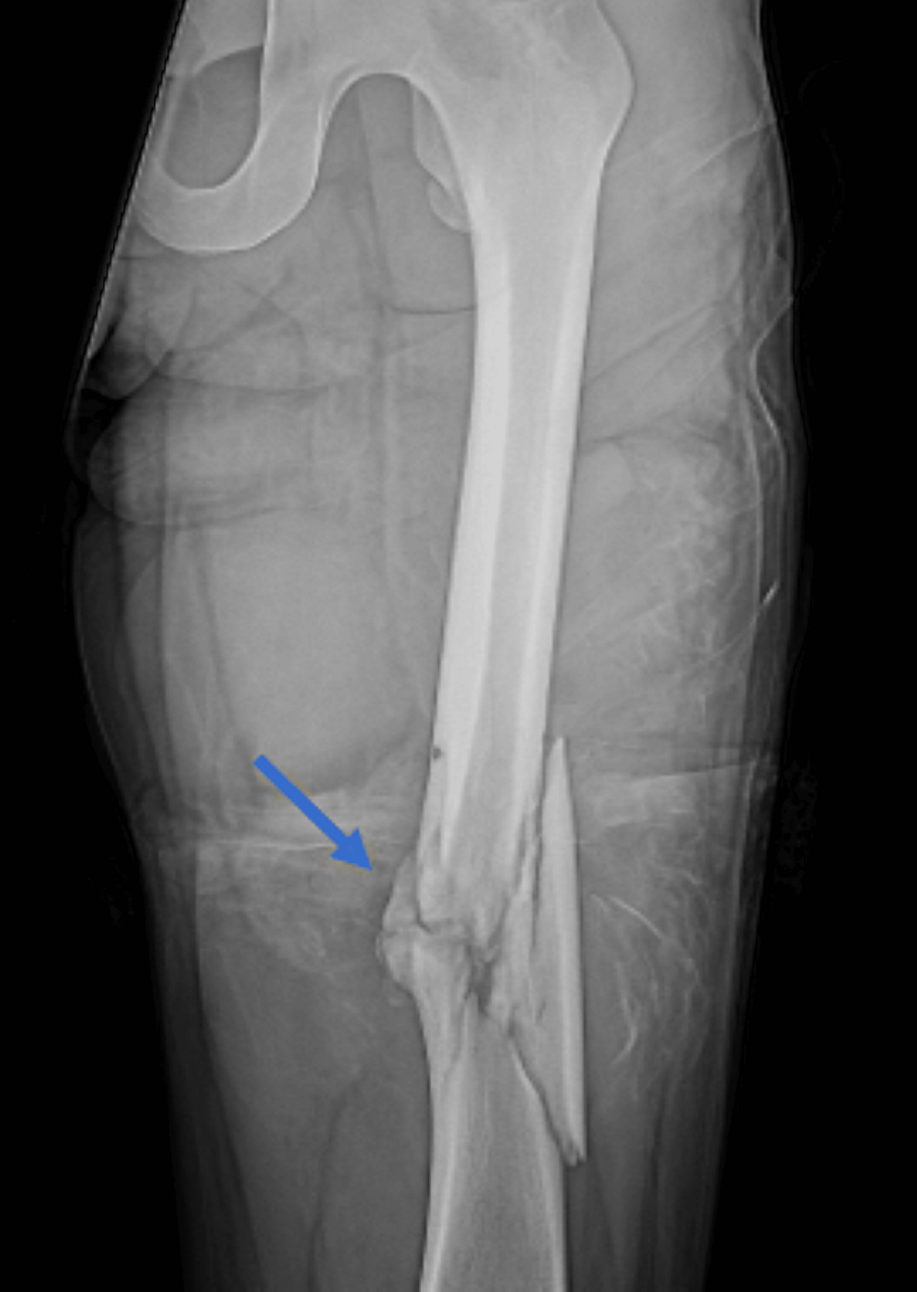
Frontal radiograph of the femur demonstrates a comminuted diaphyseal fracture involving the distal third of the femoral shaft (blue arrow)
